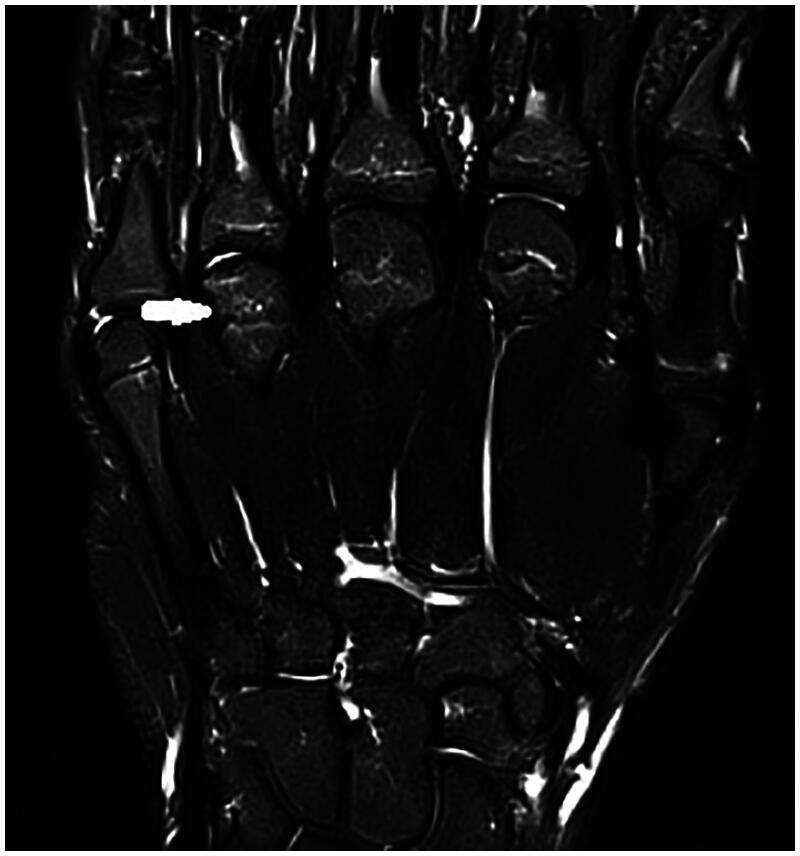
Third MRI January 2023. Apparition of signal abnormality of the fourth metacarpal head in the MRI T1 weighted images.
